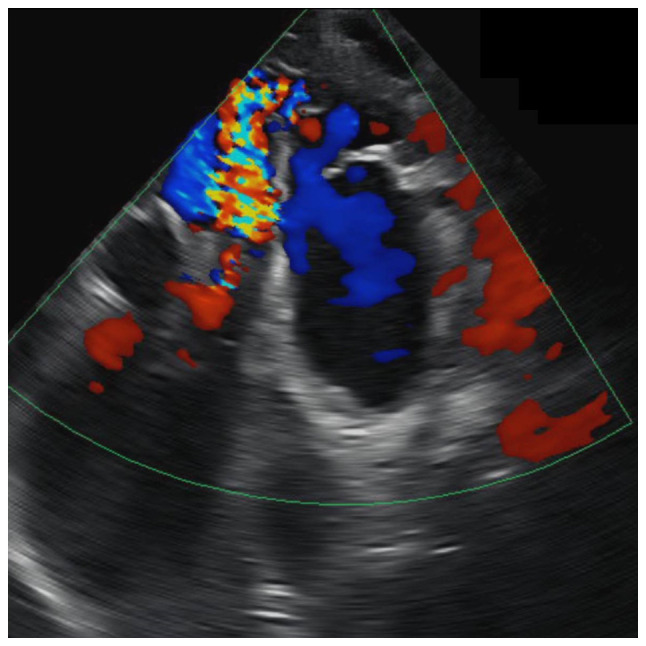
Transthoracic echocardiograph of the patient.
